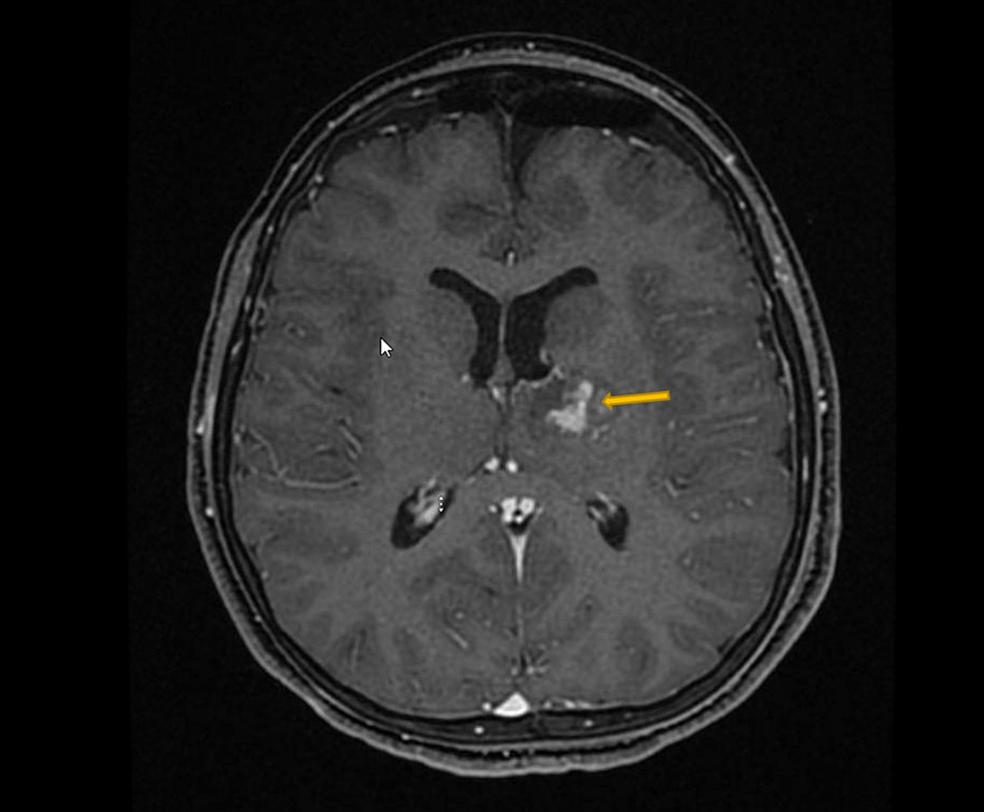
Postoperative T1-weighted MRI with contrast The image shows the residual tumor and resolution of the mass effect on the third ventricle.
